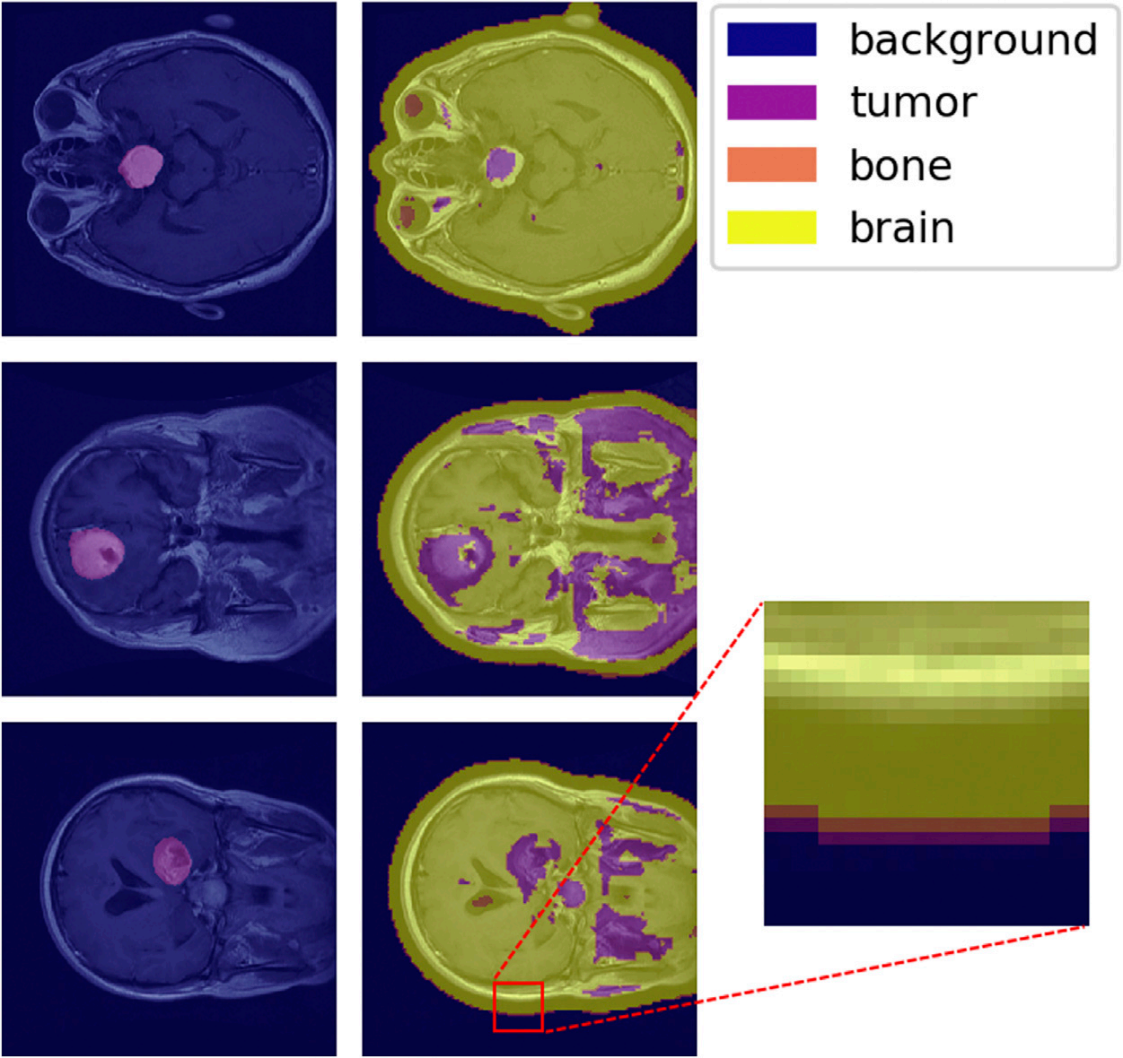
The MAC-CNN’s category decisions applied in a sliding-window manner to some full brain scans. The first column contains raw images with the expertly annotated mask (“tumor”) highlighted, while the second column contains raw images overlaid with the results from the MAC-CNN. The MAC-CNN is effective at detecting tumor regions, but often picks up extraneous noise. The network also appears to exhibit knowledge transfer from the knee X-rays, as it recognized bone textures around the perimeter of the skull that it learned from the knee X-rays.
